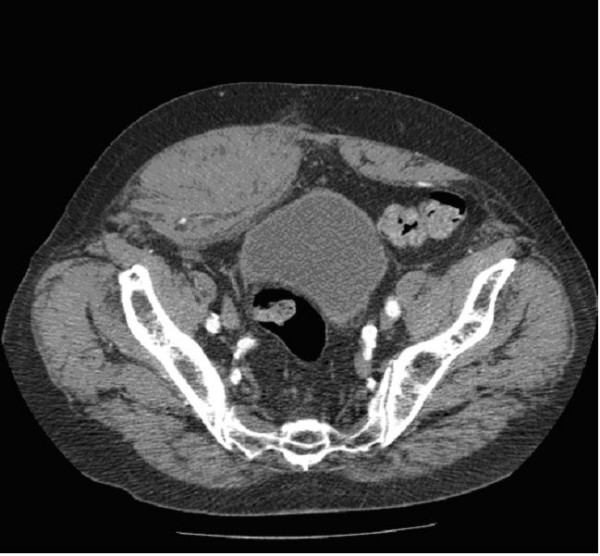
Computed tomography showing a right inferior rectus sheath hematoma.
